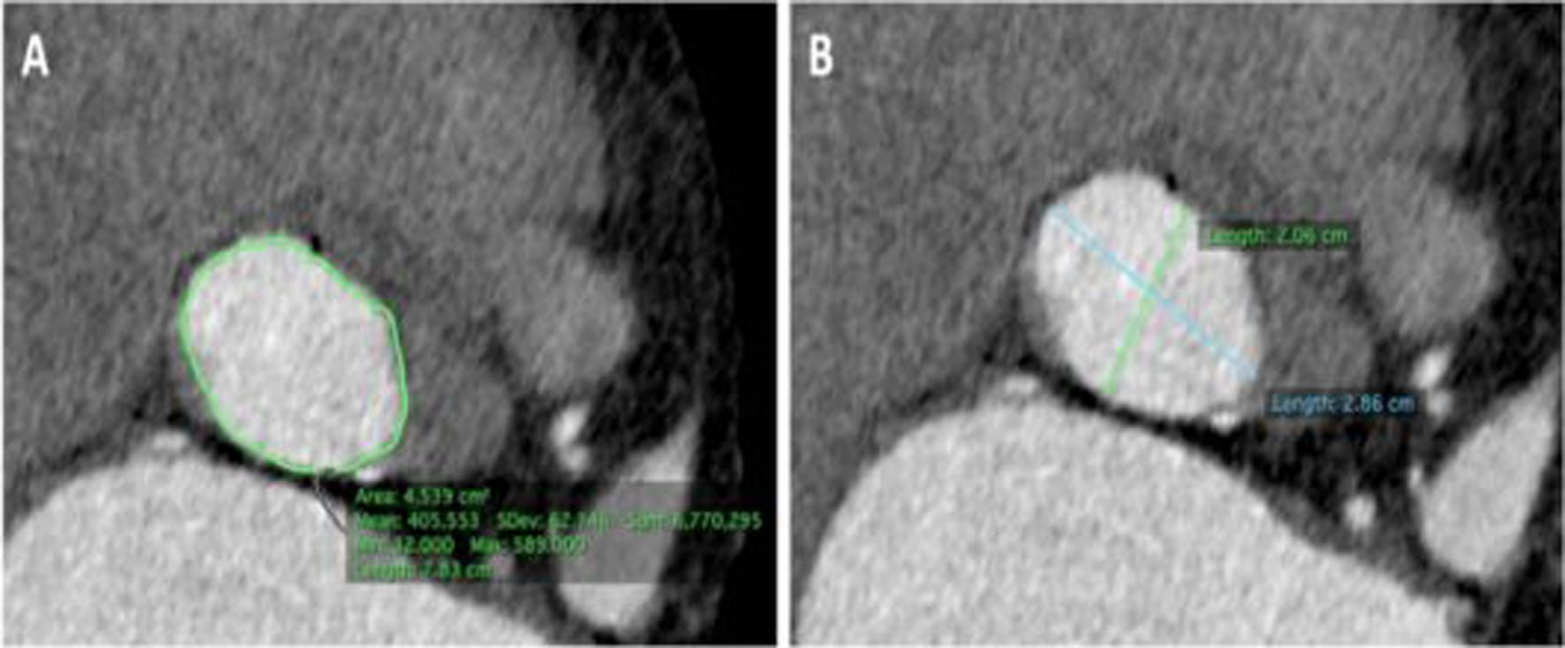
CT-derived measurements of the aortic valve annulus
